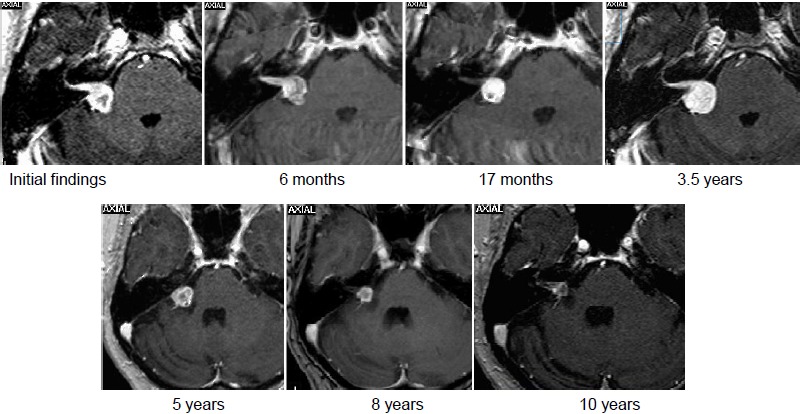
The MRI follow-up controls (T1-w + CM) show an inhomogeneous contrast enhancement of the tumor before therapy. After 6 months, minimal shrinking with slightly reduced contrast enhancement. After 17 months, again increased contrast enhancement with unchanged tumor size. After 3.5 years again tumor growth to 2.1 cm³. After second radiosurgery with 12 Gy margin dose (80%) again central contrast decrease and reduction of the tumor size to 0.12 cm³.
